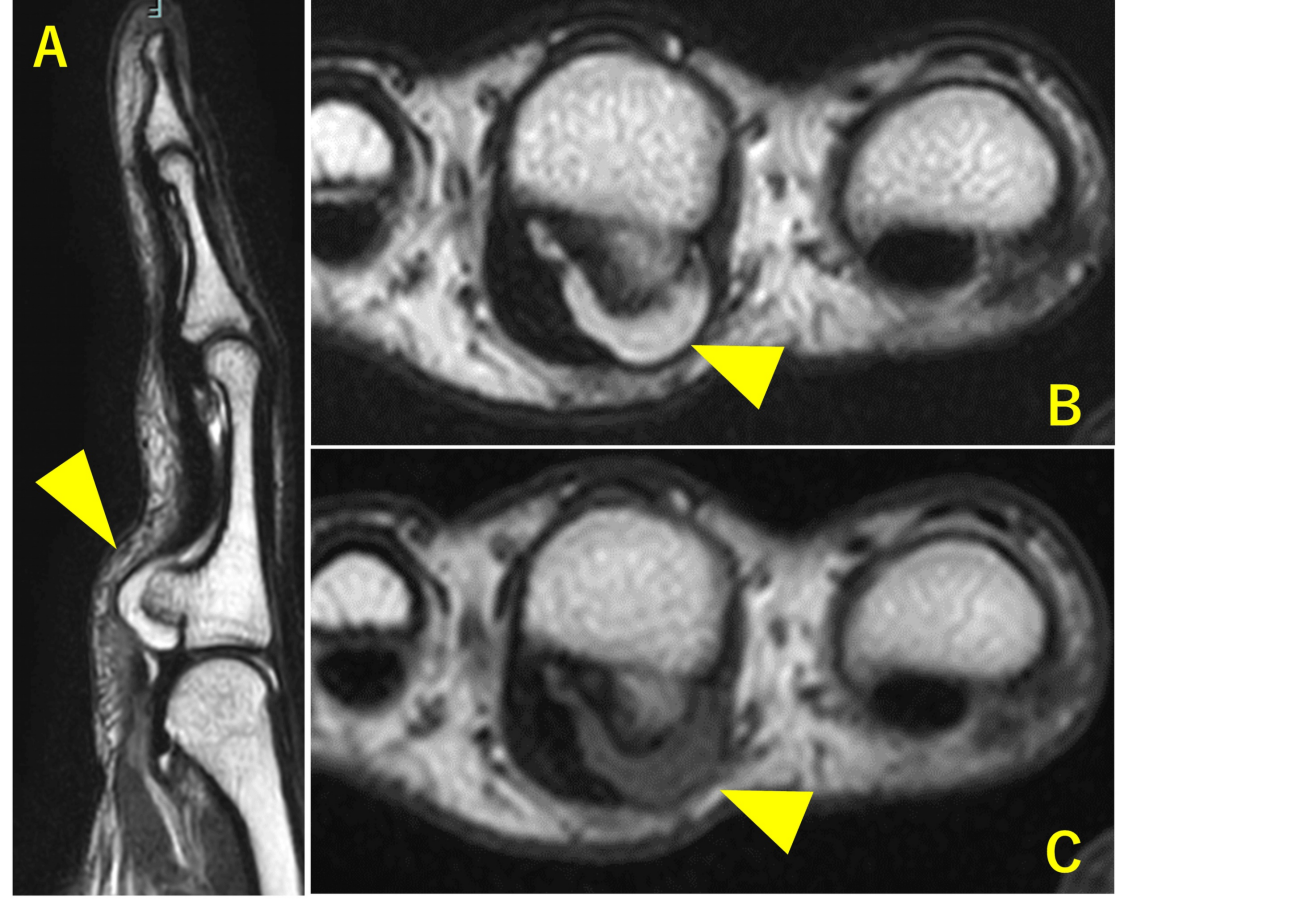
Magnetic resonance imaging. (A) T2-weighted imaging, sagittal view. (B) T2-weighted imaging, axial view. (C) T1-weighted imaging, axial view. Bone marrow continuity and a thin cartilage cap covering the bony prominence are visible.
